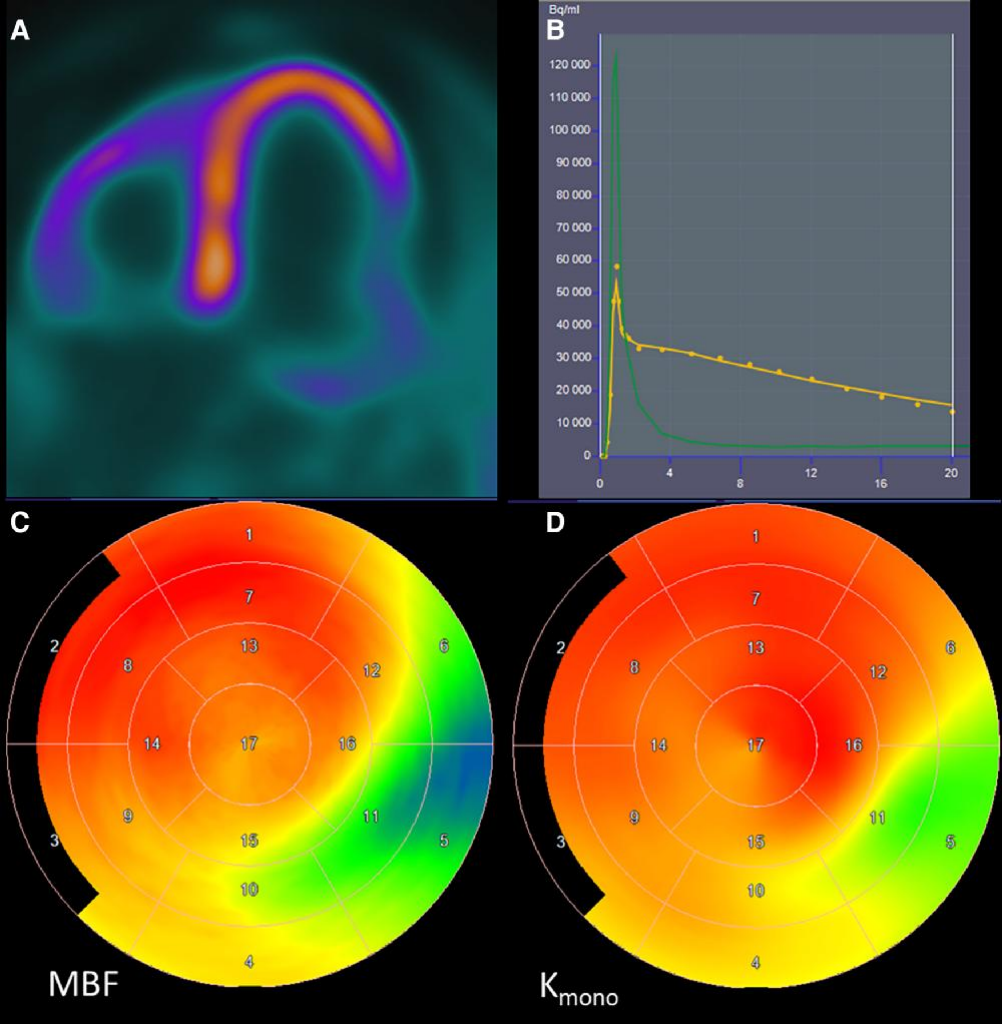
Evaluation of myocardial oxidative metabolism and efficiency of external work in a patient with ischaemic cardiomyopathy. PET image shows ^11^C-acetate accumulated in the myocardium in proportion to blood flow during the first minutes after injection (*A*). ^11^C-acetate is trapped in the myocytes as ^11^C-acetyl coenzyme A, which is metabolized in the mitochondrial tricarboxylic acid cycle, and radioactivity is cleared from the myocardium as ^11^C-carbon dioxide. The rate of ^11^C-clearance during 20 min is monoexponential (K_mono_) that is linearly related to myocardial oxygen uptake (yellow line in *B*). (*C*) Map of resting myocardial blood flow and (*D*) Kmono in the left ventricle myocardium are shown. Note reduced blood flow and Kmono in the area of infarct scar in the lateral wall. Myocardial external efficiency can be calculated as the ratio of cardiac work (cardiac output per myocardial mass times mean arterial pressure) and myocardial oxygen uptake. Cardiac output can be measured by ultrasound, cardiac magnetic resonance, or a recently developed method based on radioactivity concentration in the left ventricle cavity.^91^
